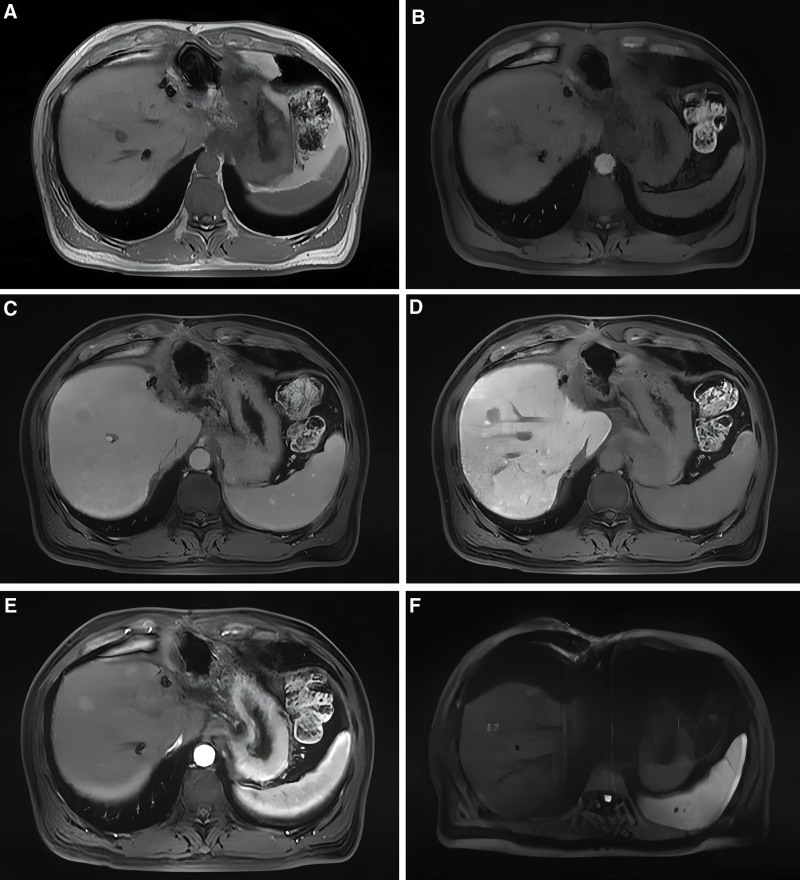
New hepatocellular carcinoma in segment SVII was detected during a 5-mo postoperative abdominal MRI follow-up. (A) A slightly shorter rounded T1 signal shadow is observed in the hepatic segment SVII; (B) a round enhancing shadow is visible in the arterial phase; (C) enhancement diminishes in the delayed phase; (D) low signal in the hepatobiliary phase; (E) enhancement decreases in the portal venous phase; (F) no prominent abnormally restricted high-signal shadow is observed in DWI. MRI = magnetic resonance imaging.
